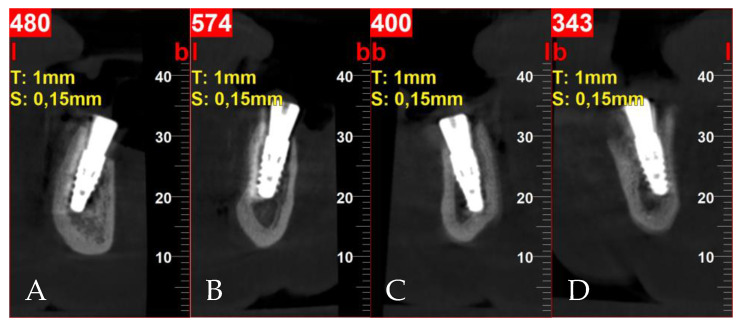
Postoperative cross-sectional CBCT scans: (A) tooth 32; (B) tooth 34; (C) tooth 42; (D) tooth 44.
